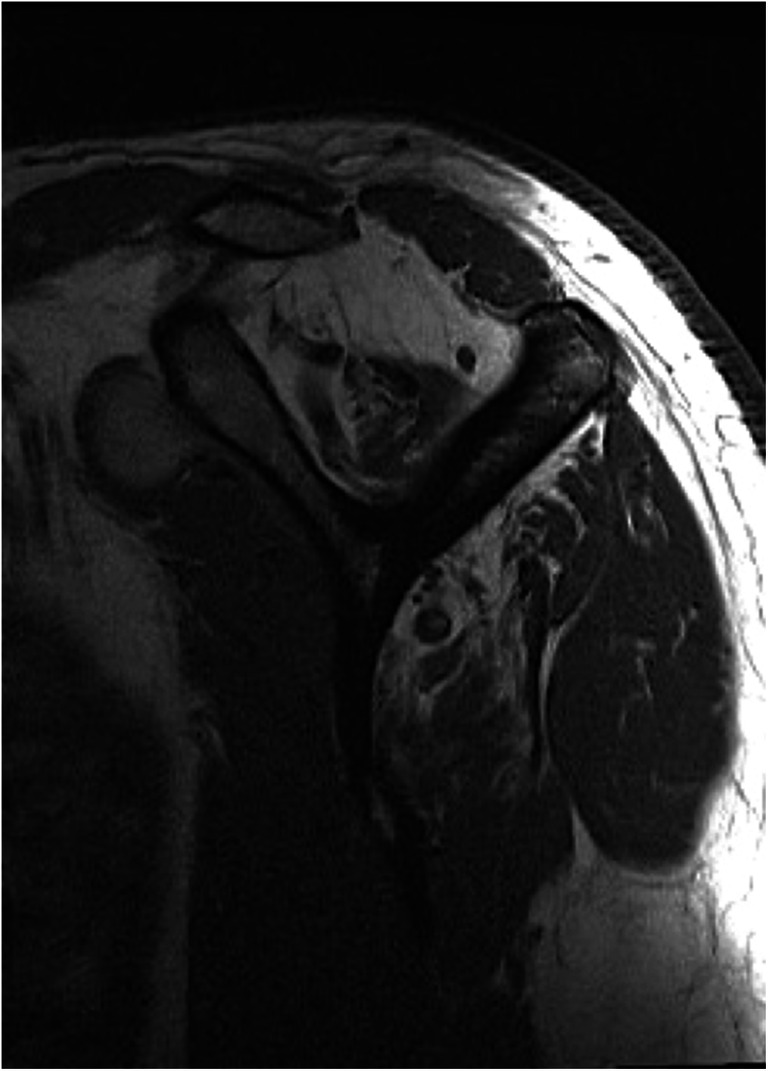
Parasagittal T1-weighted MR imaging showing advanced fatty infiltration of the supraspinatus (with atrophy) and infraspinatus muscles. Muscles of the subscapularis and teres minor show no degeneration
